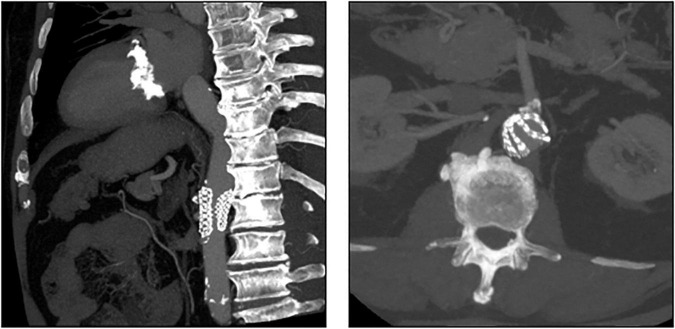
CT images of a patient with embolization of an Evolut PRO. In this case (patient number 8 from Table 5), the snare used to pull the embolized Evolut PRO further into the ascending aorta was entangled in the valve frame. The bent Evolut PRO was eventually pulled into the descending aorta where the snare could be liberated.
